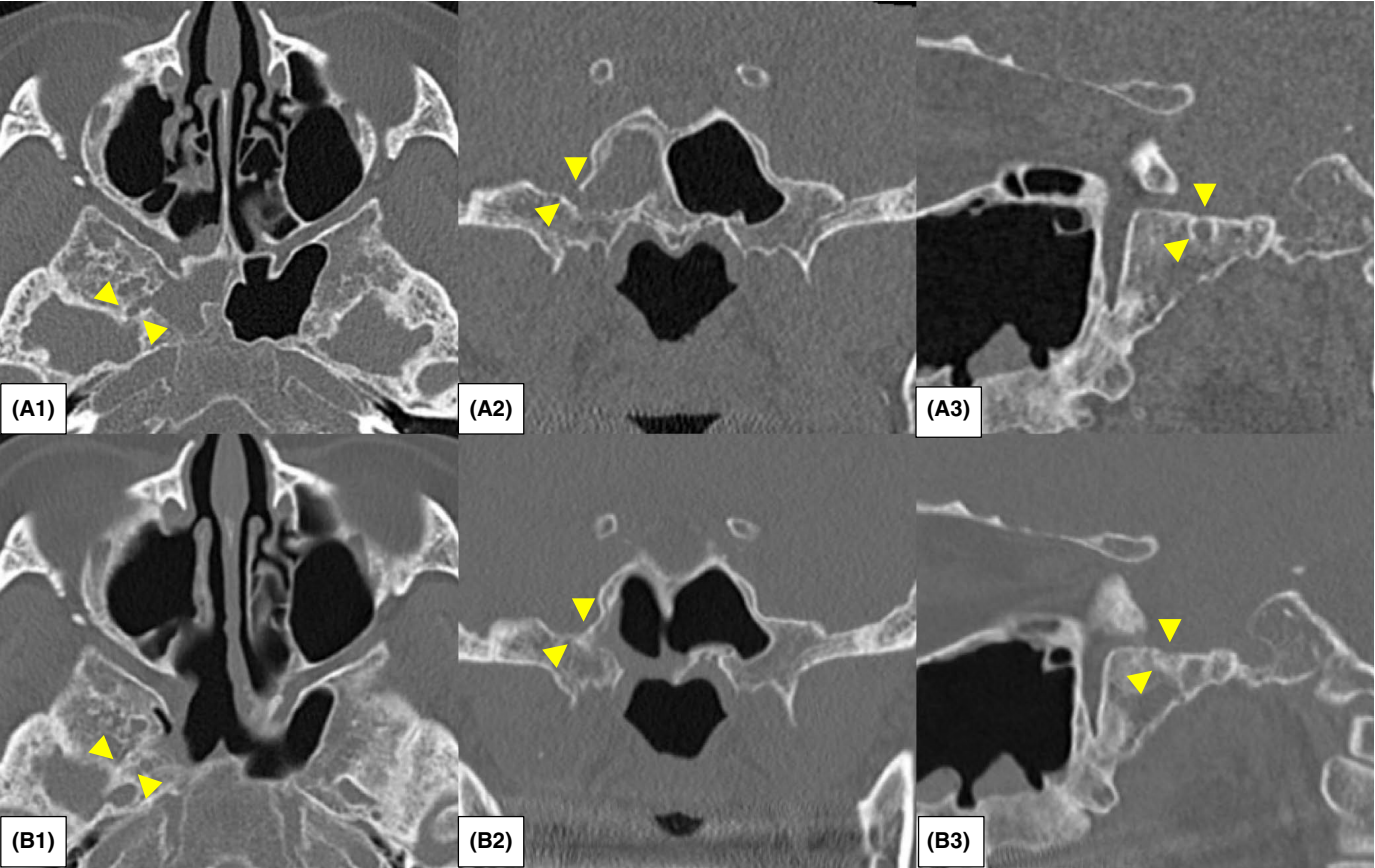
Comparison of pre‐ and postoperative CT images. (A) Preoperative high‐resolution (HR) CT images: A1, axial section; A2, coronal section; A3, sagittal section. The yellow arrowheads point to the fistula. (B) Postoperative HRCT images: A1, axial section; A2, coronal section; A3, sagittal section. The yellow arrowheads point to the fistula. The fistula is filled with bone pile
